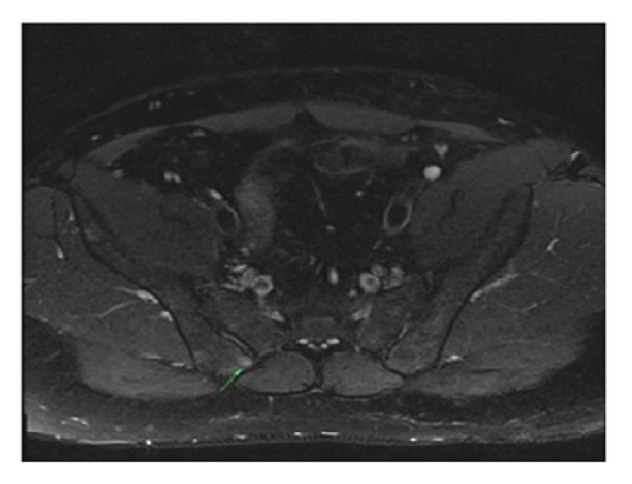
Sacroiliac joint magnetic resonance imaging was consistent with sacroiliitis at right posterior of the sacroiliac joints.
